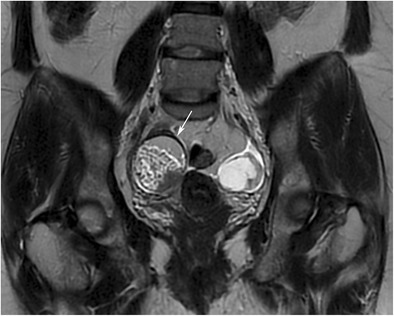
Coronal T2-weighted MR image of a 42-year-old female with mature cystic teratoma shows chemical shift artefact both inside the tumour and in the borders. A low signal intensity band (arrow) is seen on the cranial border of the cyst with a high intensity band on the opposite side
